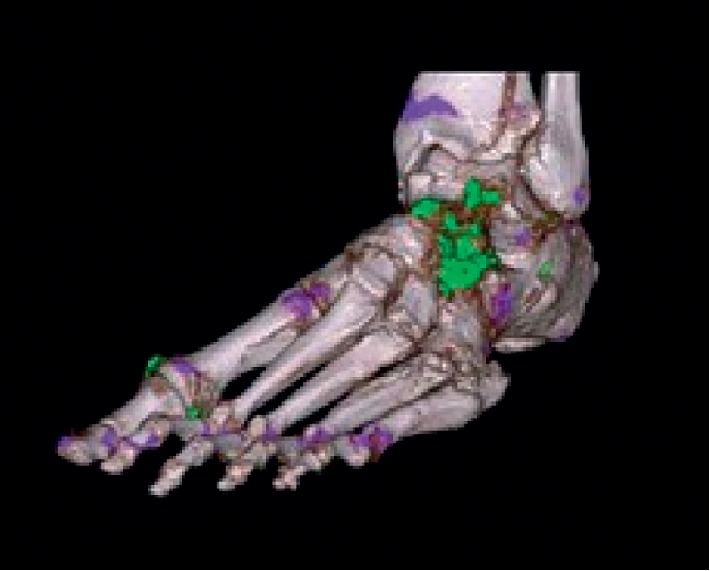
Left foot with Dual energy CT 3D-reconstruction.
